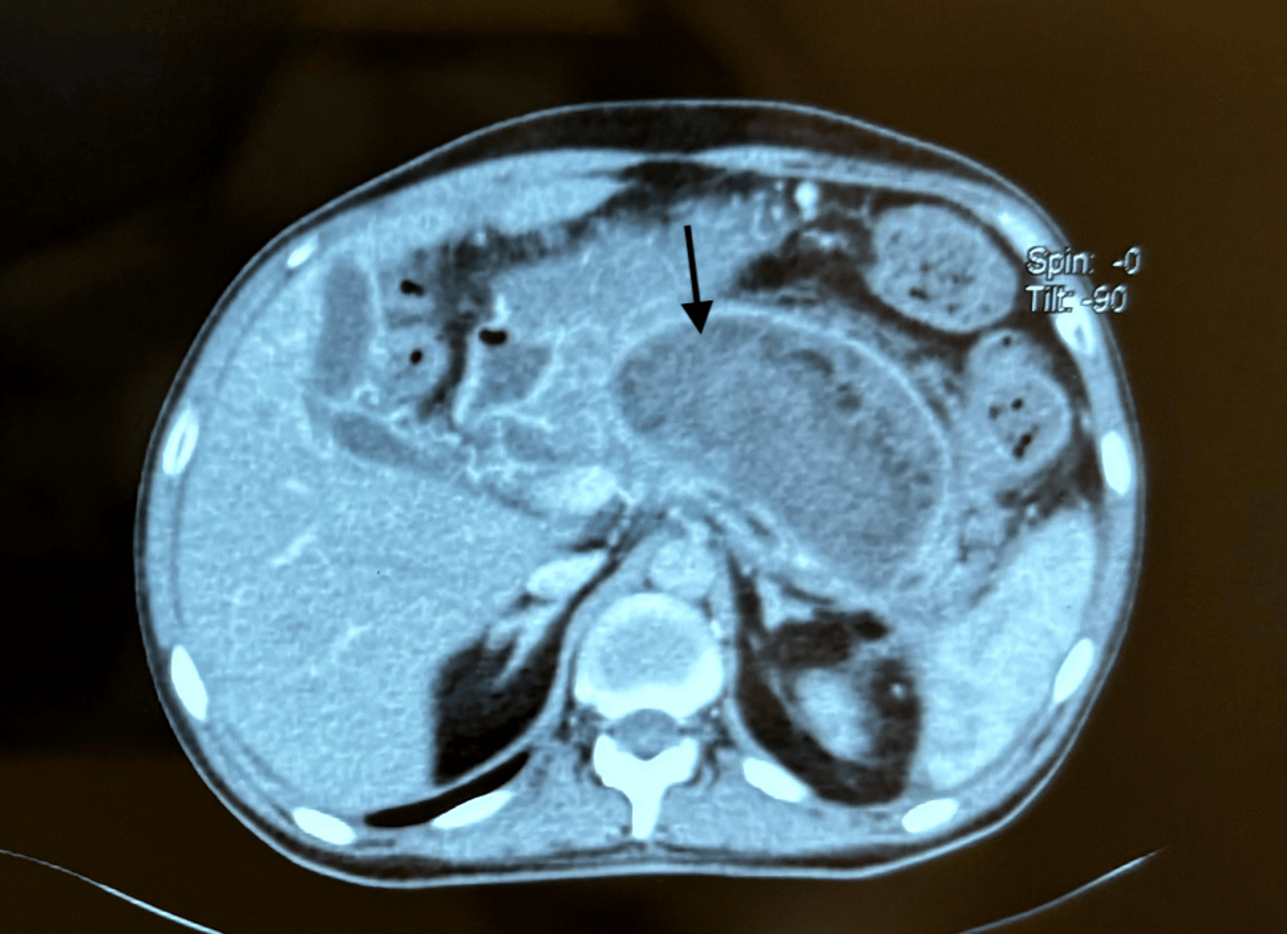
Abdominal CT scan in axial section with injection of contrast medium showing a pseudocyst of the pancreas (arrow).
